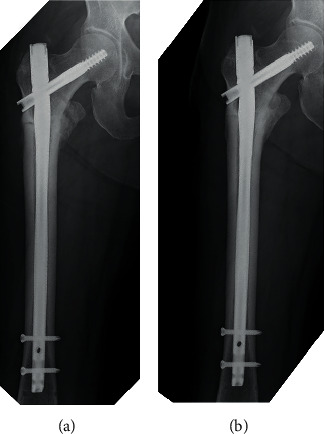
Time course of radiographs after the revision surgery. Three months after the revision surgery, callus formation is detected at the fracture site (a). At 13 months after the revision surgery, the fracture site has healed (b).
